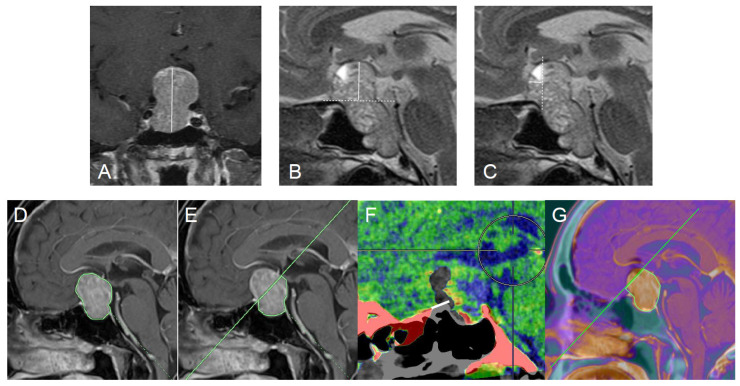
(A) Tumor height (white line) was measured on the coronal plane of MR imaging. (B) Anterior skull base–tumor top distance (white line) was measured on the mid-sagittal plane of MR imaging. This measurement represents the vertical distance from the extended line of the anterior skull base (white dotted line) to the tumor apex. (C) Tumor anterior tip-tuberculum sellae distance (white line). The white line connects the anterior tumor margin and the posterior border of the tuberculum sellae (white dotted line). (D) Total tumor area measured on mid-sagittal MR imaging using the Synapse Vincent workstation. (E) Area of the TSA, defined as the region below the line connecting the anterior nasal spine and the tuberculum sellae. (F) Planum removal distance (white line). This fusion image combines pre- and postoperative CT scans to delineate the extent of planum sphenoidale removal. (G) Area of the mTSA, defined as the region below the line connecting the anterior nasal spine and posterior edge of the resected planum sphenoidale.
